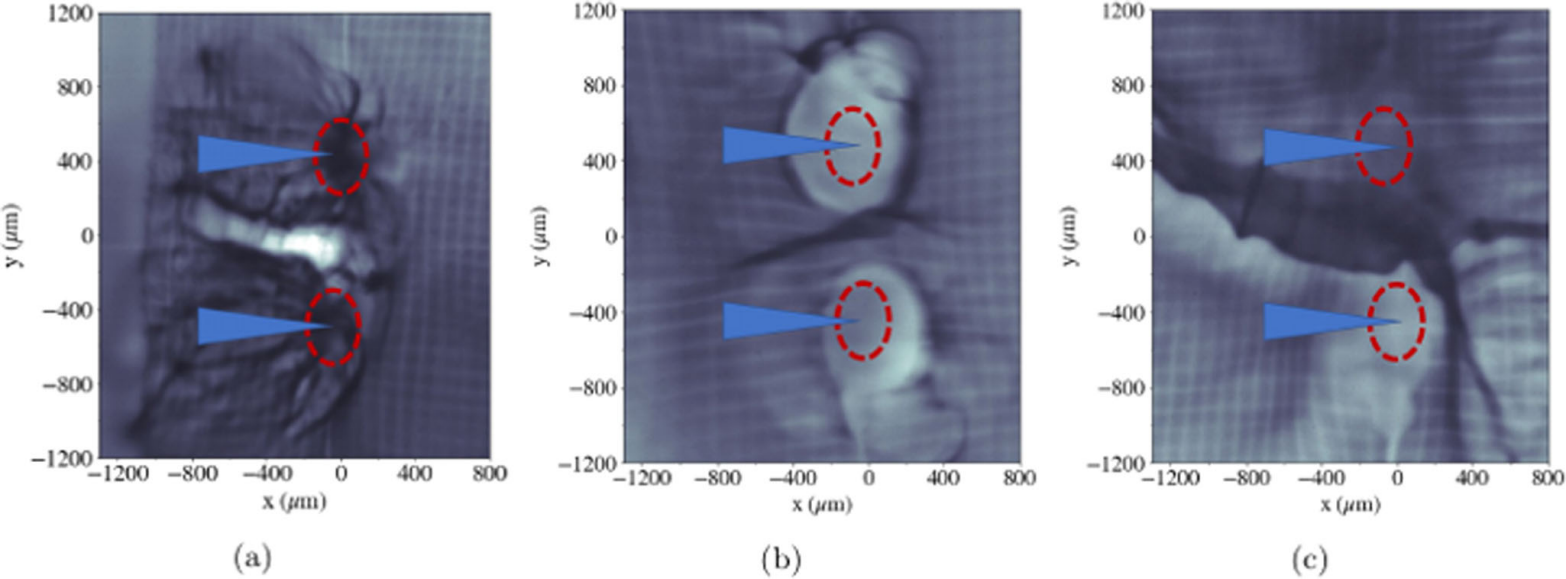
Proton radiographs from probing at 45° to the target
